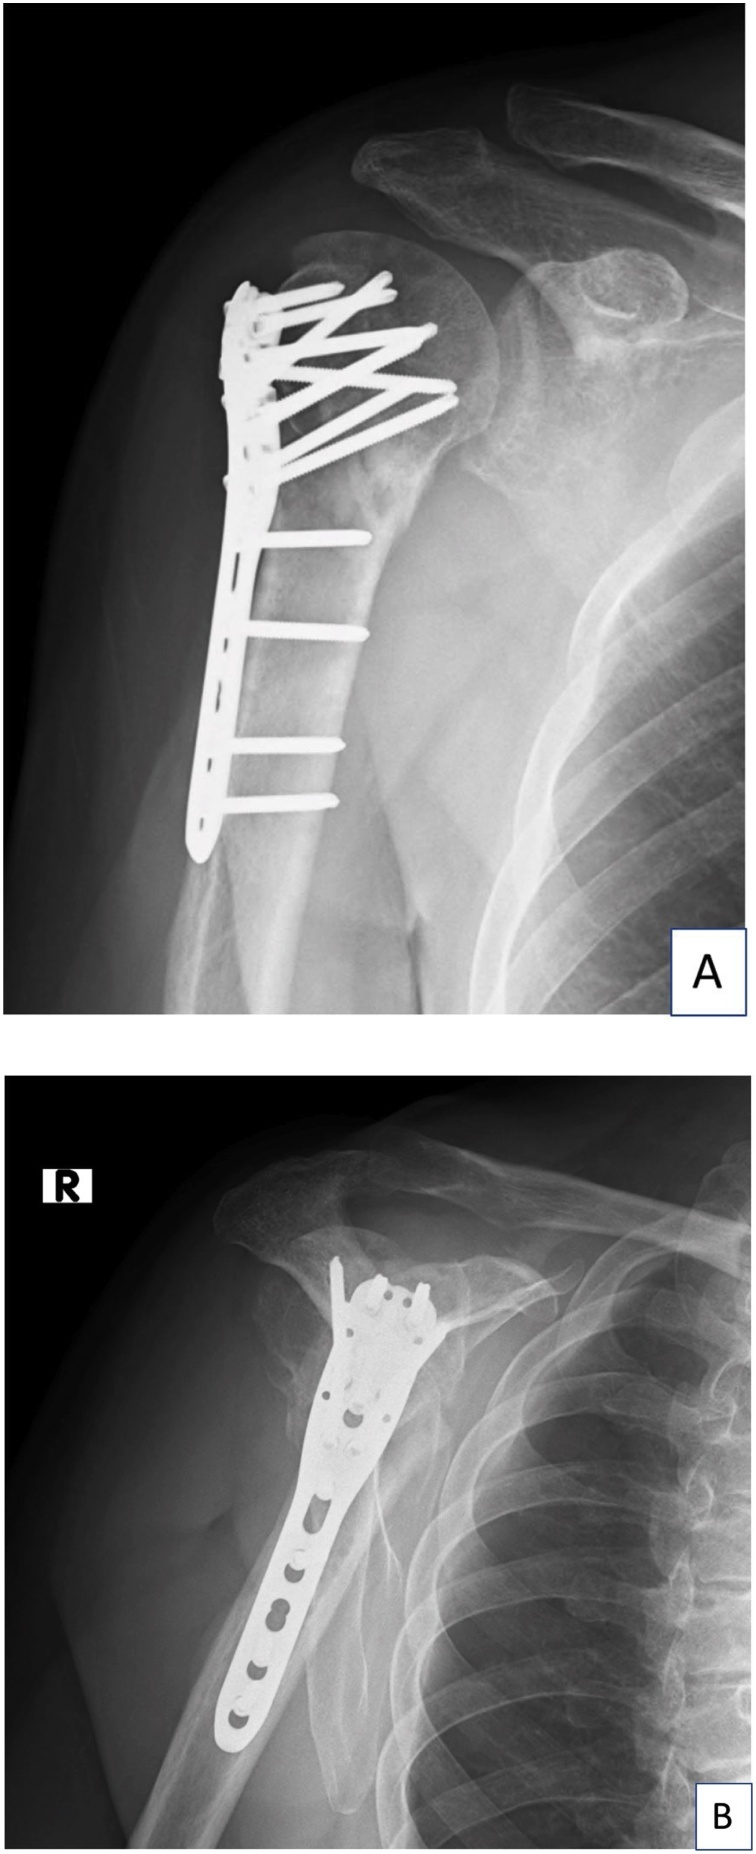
Radiographic imaging: (A) anteroposterior view of right shoulder, and (B) transcapular view of right shoulder at 6-month follow up.
